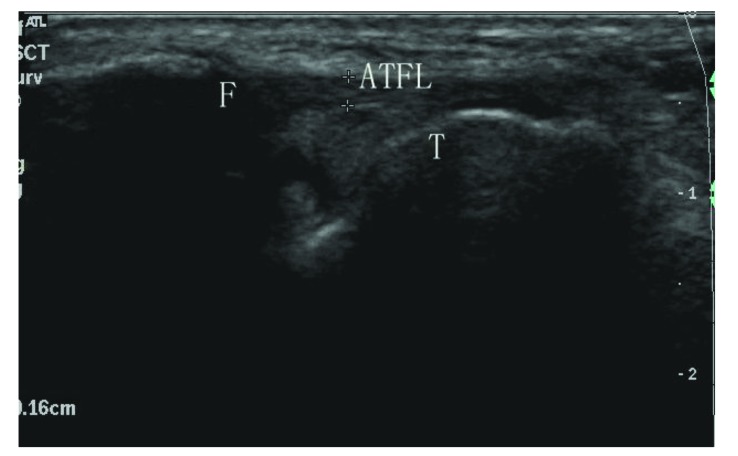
Sonogram shows a normal anterior talofibular ligament (type I). ATFL: anterior talofibular ligament; F: fibula; T: talus.
